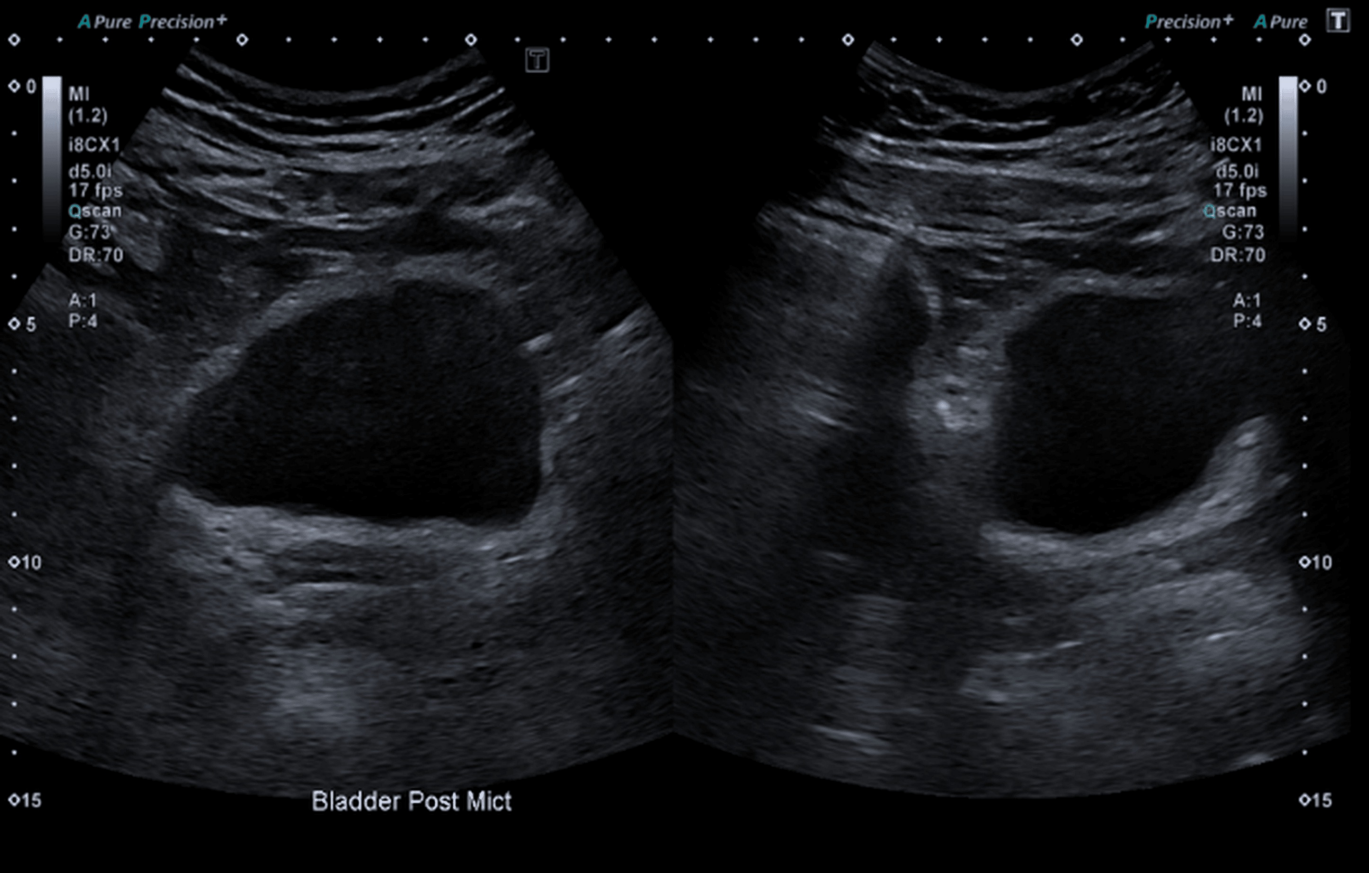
Ultrasound of the urinary bladder showing a normal appearance, although the bladder has a large post-void residual volume of 138 cc
